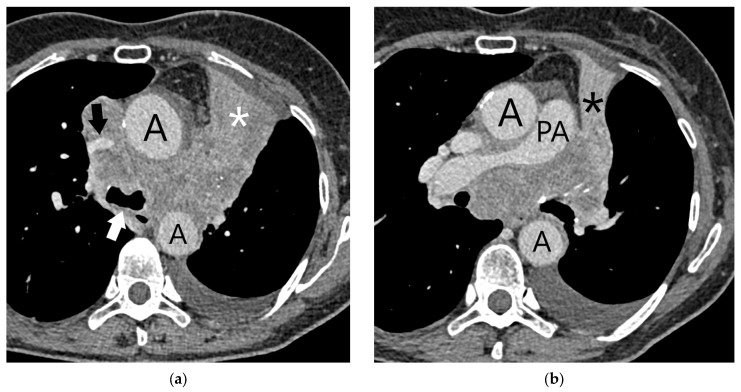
T4 category: Axial contrast-enhanced CT scan of the chest at the level of carina (a) and the main pulmonary artery (b) shows a T4 category upper-left lobe lung cancer with invasion of the aorta (A), intrapericardial segments of pulmonary arteries (PA), carina (white arrow), and the superior vena cava (black arrow). Additionally, there is upper-left lobe atelectasis (asterisk) and left-sided pleural effusion.
